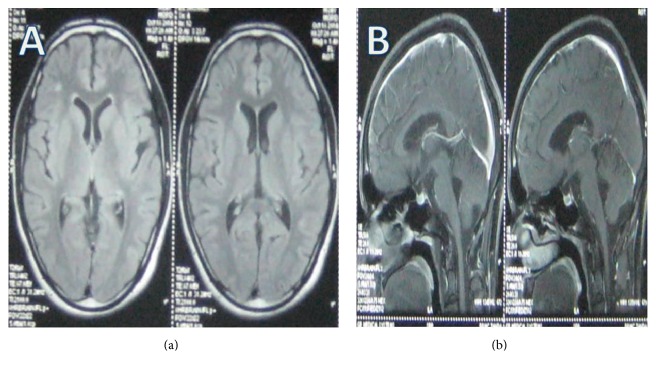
Follow-up MRI 6 months later showing (a) axial FLAIR images showing partially resolved white matter periventricular and subcortical hyperintensities. (b) After contrast, no brainstem meningeal enhancement is seen.
